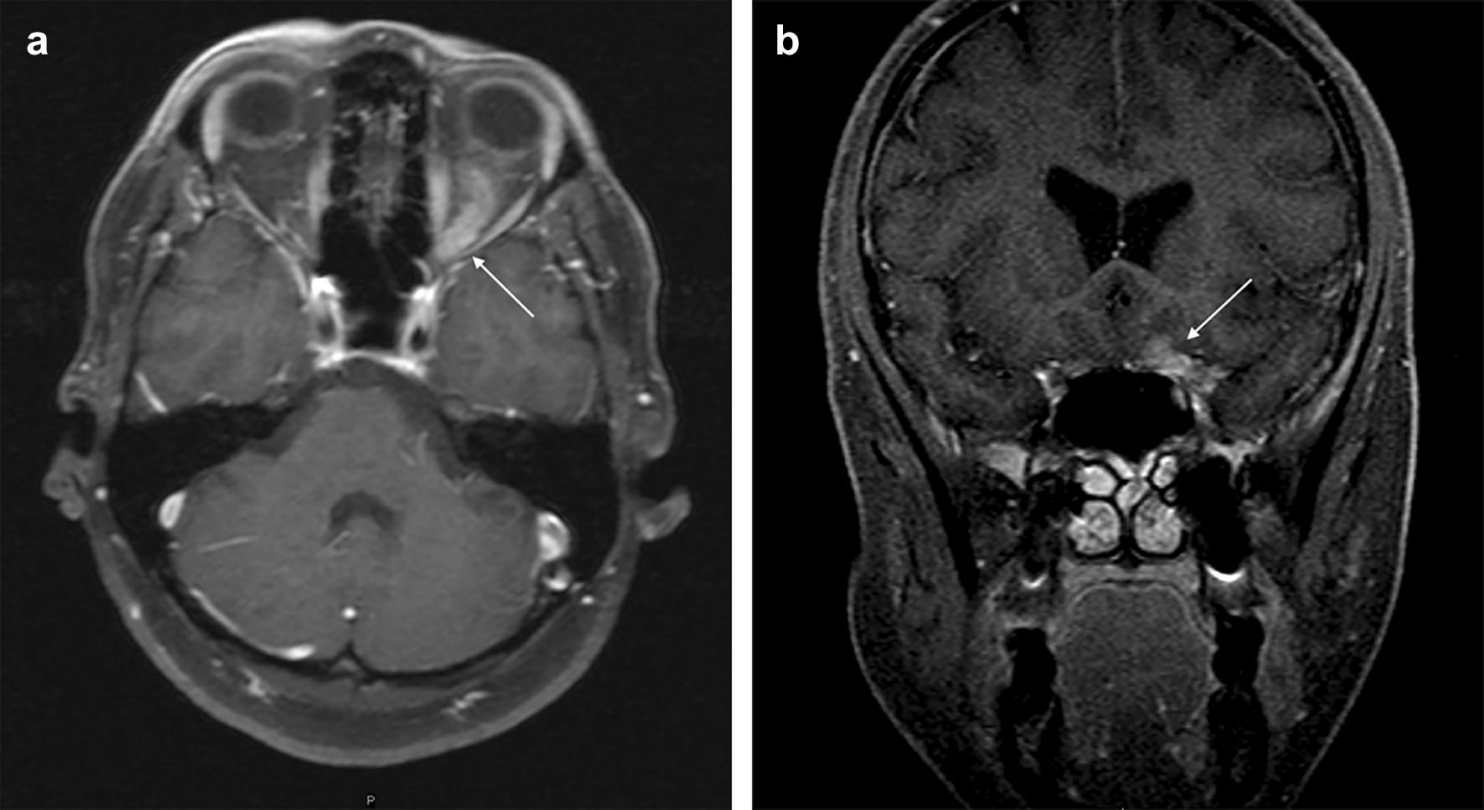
Sarcoidosis engaging optic nerves. (a) Post-contrast T1-weighted axial image shows diffuse enhancement and thickening of the left intra orbital optic nerve sheath in a 48-year-old female presenting with progressive vision loss first in left eye concerning for optic glioma. Upon biopsy a diagnosis of definite optic sarcoidosis was made. A few months later, she presented with progressive vision loss in the right eye. Receiving immunotherapy, the vision has been stable for 13 years. (b) Cor T1 fs Gd Shows intracranial extension of contrast enhancement around the left optic nerve.
